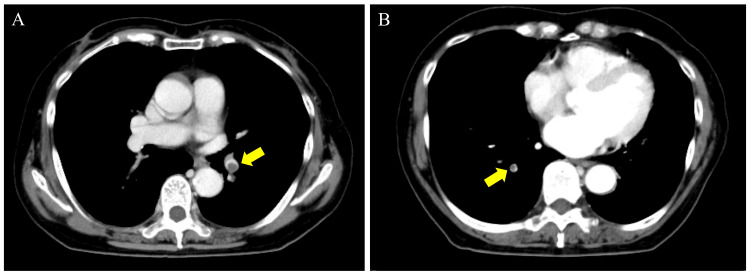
Contrast-enhanced CT of the chest Contrast-enhanced CT of the chest showing thrombi (yellow arrow) in the lower lobe branch of the left pulmonary artery (A) and in the lower lobe branch of the right pulmonary artery (B).
